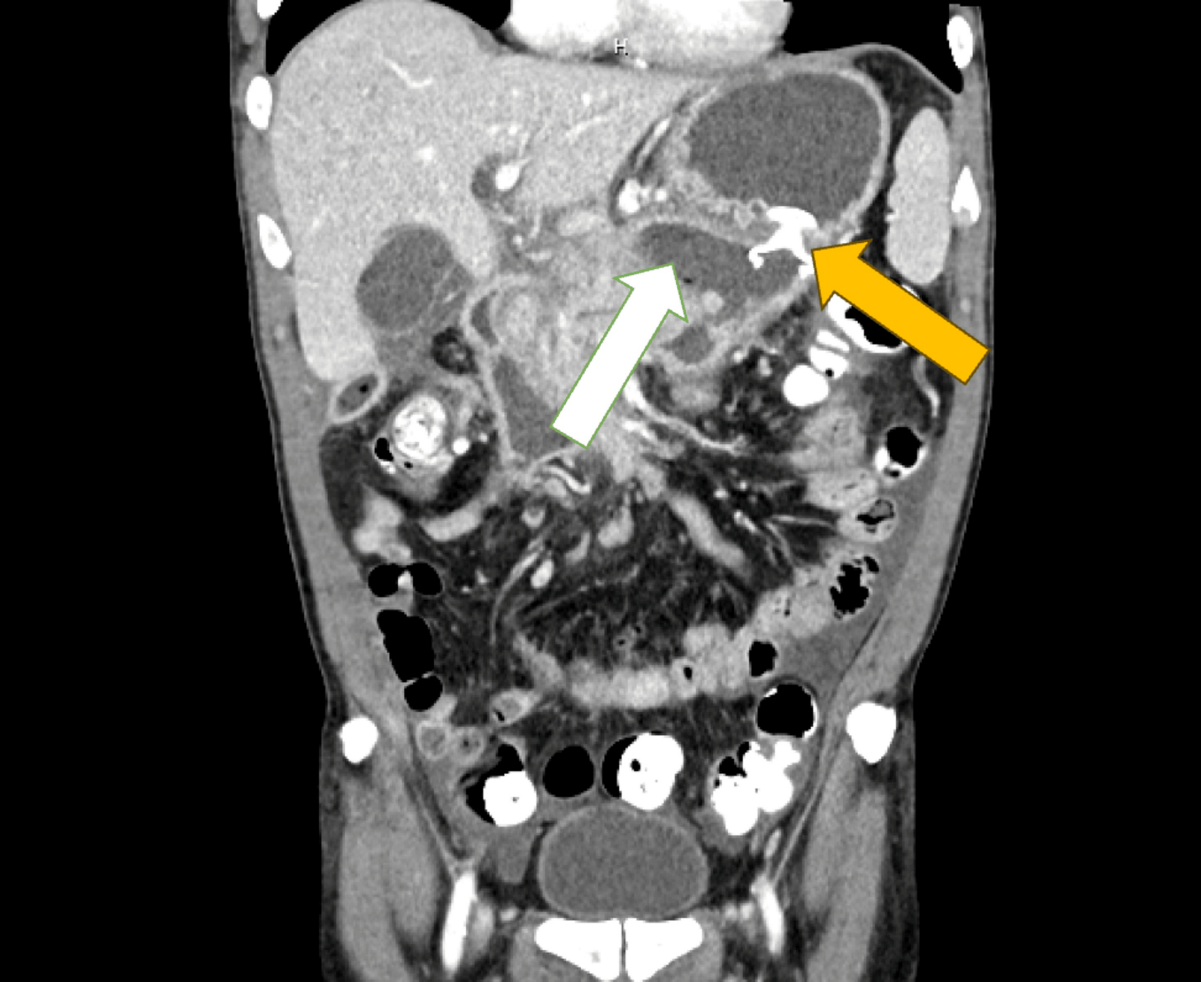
Coronal computed tomography image (contrast-enhanced) The image shows a large fluid-filled collection in the region of the pancreas, consistent with a pancreatic pseudocyst (white arrow). It has well-defined borders and adjacent bowel loops with a stent in place (yellow arrow).
